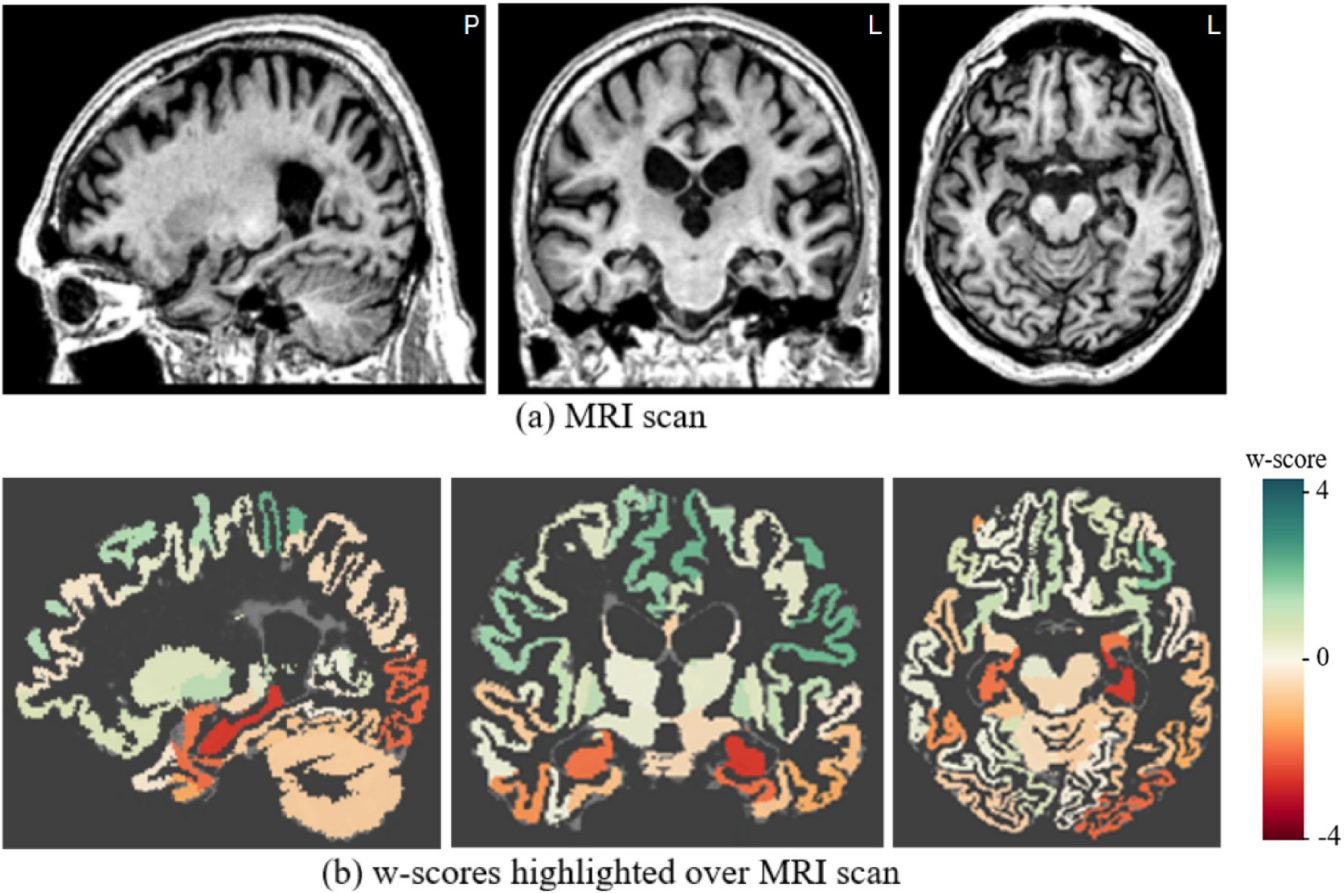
Single-subject's (a) MRI scan and (b) overlaid volumetric w-score map for the ADNI Alzheimer’s patient ID 6849 with mild dementia.
